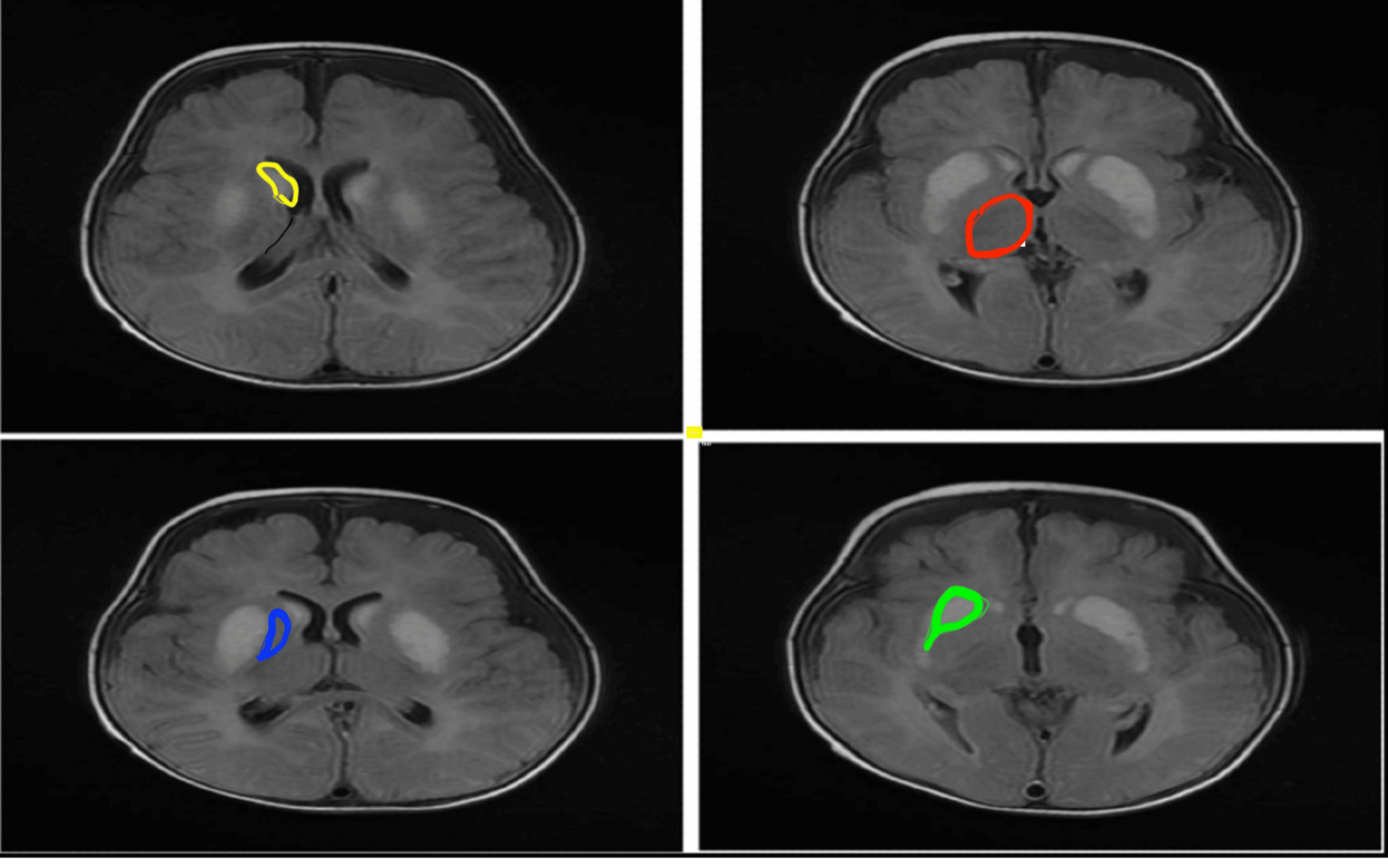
Brain MRI demonstrates basal ganglia (a) Caudate nucleus (yellow); (b) thalamus (red); (c) globus pallidus (blue); (e) putamen (green)
